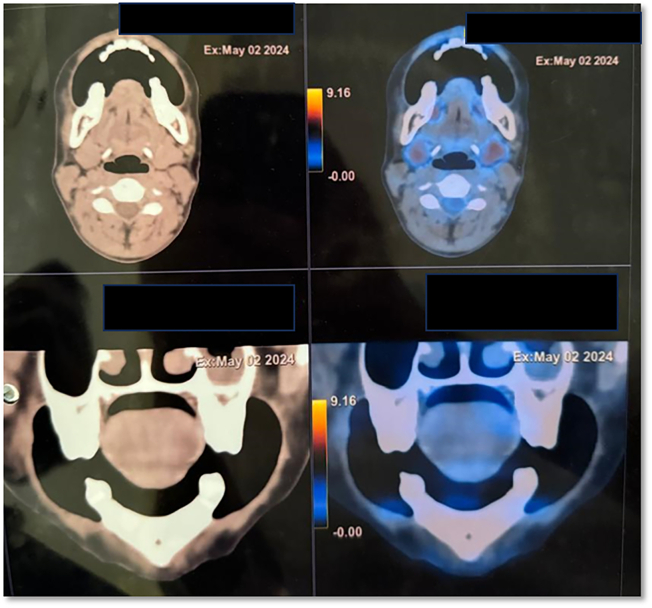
PET-CT - no FDG avid lesion noted in right buccal mucosa.
